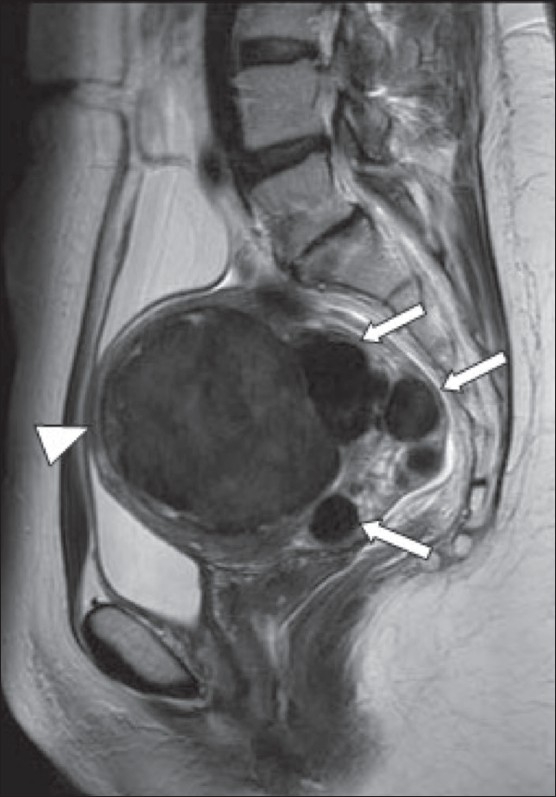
A 43-year-old woman with menorrhagia. Sagittal T2W MRI image shows multiple intramural fibroids (arrows); the largest (arrowhead) lying anteriorly measures 8.5 cm. These show typical low-signal intensity
